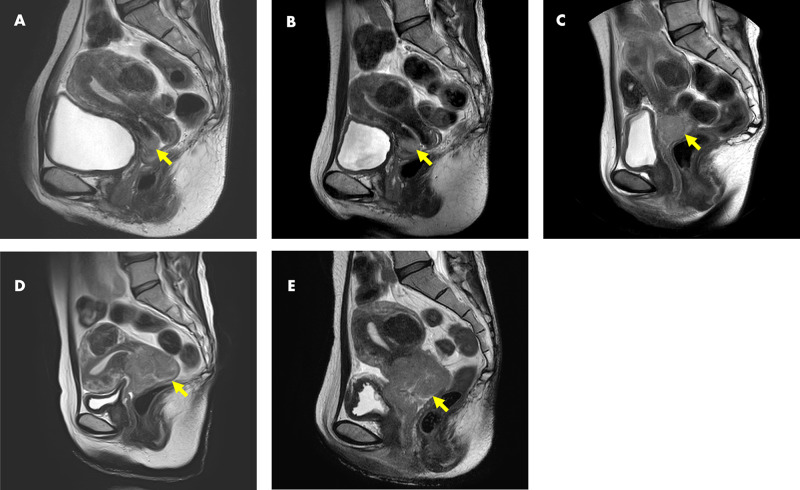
Image of magnetic resonance imaging T2 sagittal: (A) first time (Day 0), (B) Day 126, (C) Day 216, (D) Day 427, and (E) Day 512.
